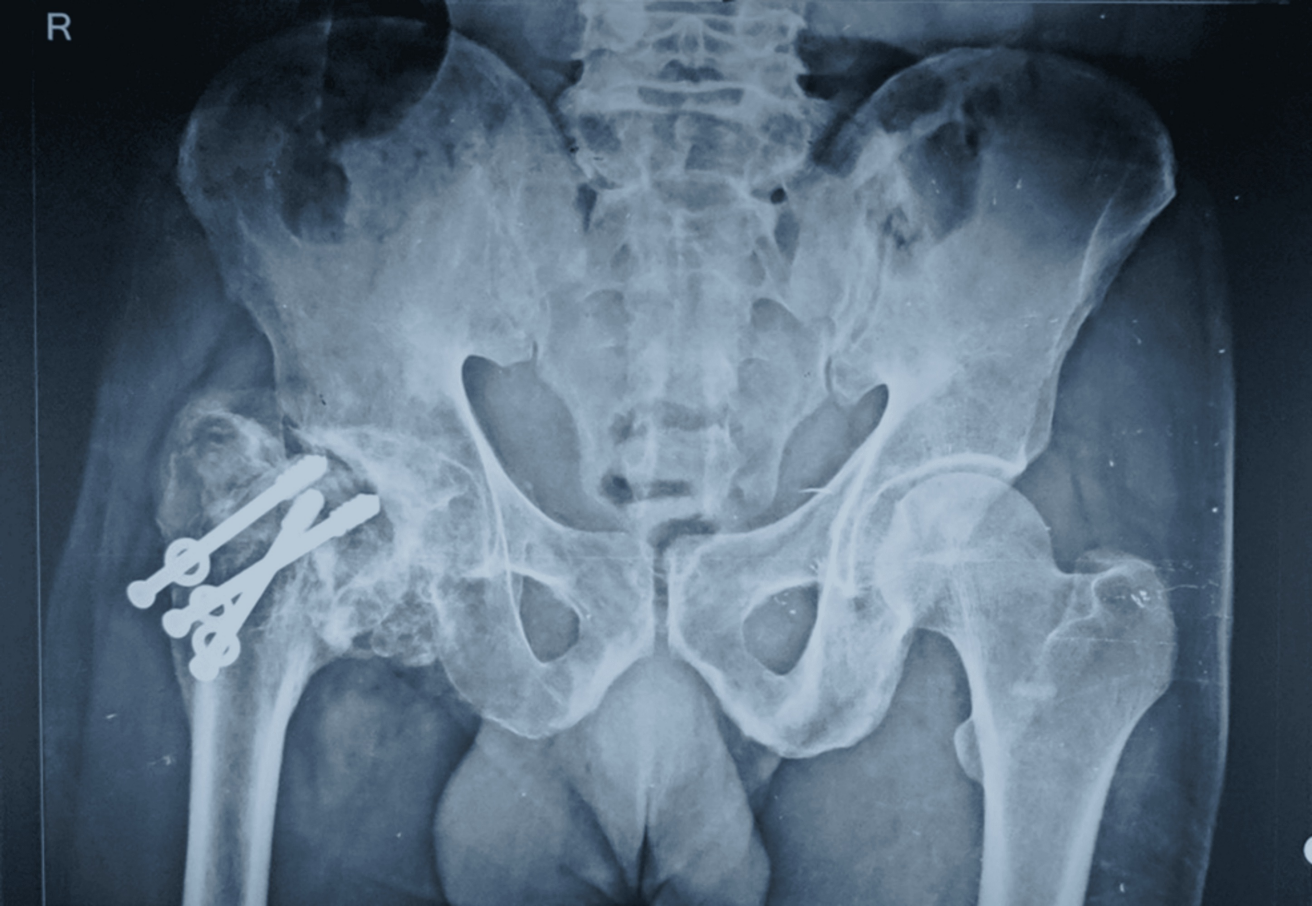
Case 2: Pre-operative X-ray of a 56-year-old male with secondary osteoarthritis of the right hip and implant in situ.
